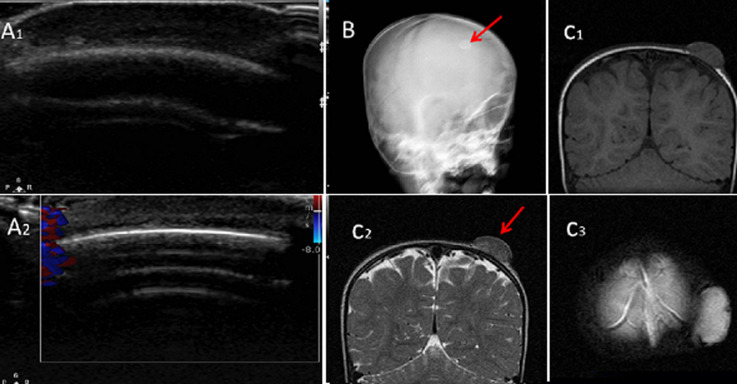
A) (1,2) ultrasound examination findings at the area of interest; B) skull radiography; C) (1,2,3) magnetic resonance imaging (MRI) scan (T1W, T2W and flair sequences)
